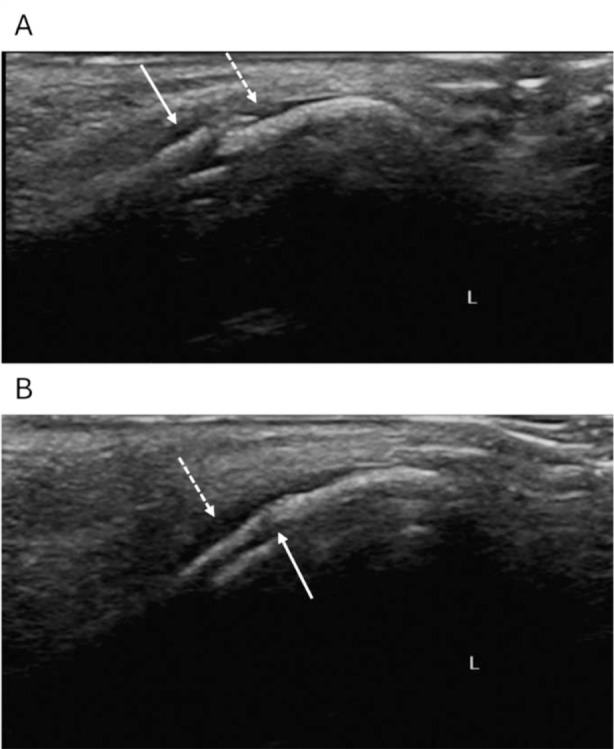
Ultrasound image of a 22-year-old male patient with simple linear nasal bone fracture. Pain and swelling of the right orbit after blunt trauma. A: The hyperechoic zone of bone cortex is interrupted continuously and separated by dislocation (solid line arrow) and a small patchy anechoic area can be seen around the fracture (dotted line arrow). B: After reduction of nasal bone fracture, the fracture is well aligned (solid arrow) and only a narrow band of anechoic area (dotted arrow) can be seen around it.
